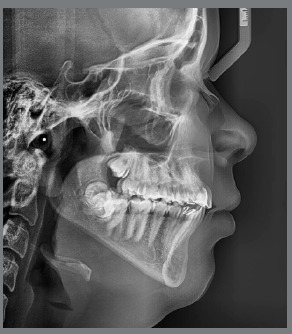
26-month retention cephalometric radiograph.
